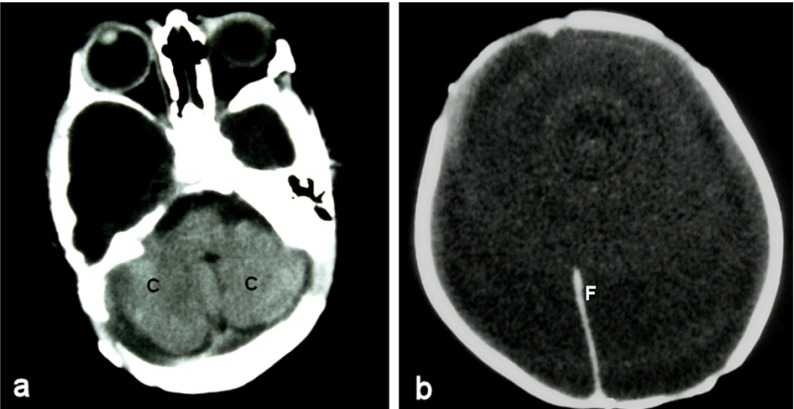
CT images of 2 days old newborns brain. (
